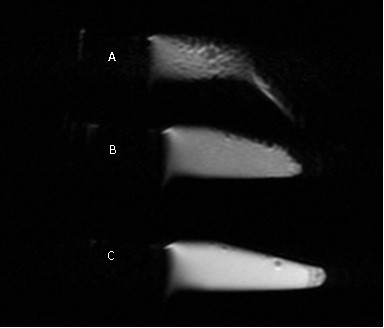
MR imaging of MGC803 cells and HDF cells. A: MGC803 cells with anti-BRCAA1-FMNPs. B:HDF cells with anti-BRCAA1-FMNPs. C: MGC803 cells with only FMNPs.
